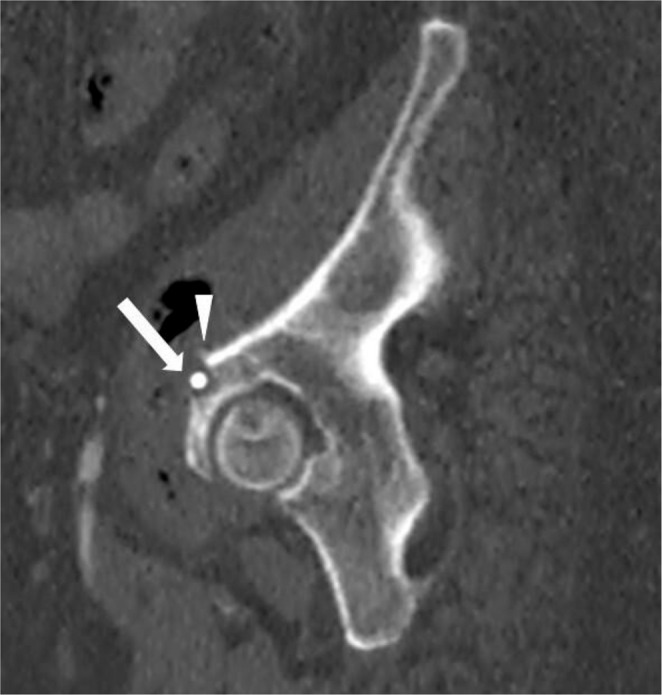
Sagittal contrast-enhanced CT of the pelvis in bone window. A migratory stent is seen causing focal erosion in the anteromedial aspect of the acetabular roof, with sharp margins and a smooth contour, extending into the central medullary portion of the iliac bone (arrow). A single-layered, linear periosteal reaction is observed in a small area immediately adjacent (arrowhead)
